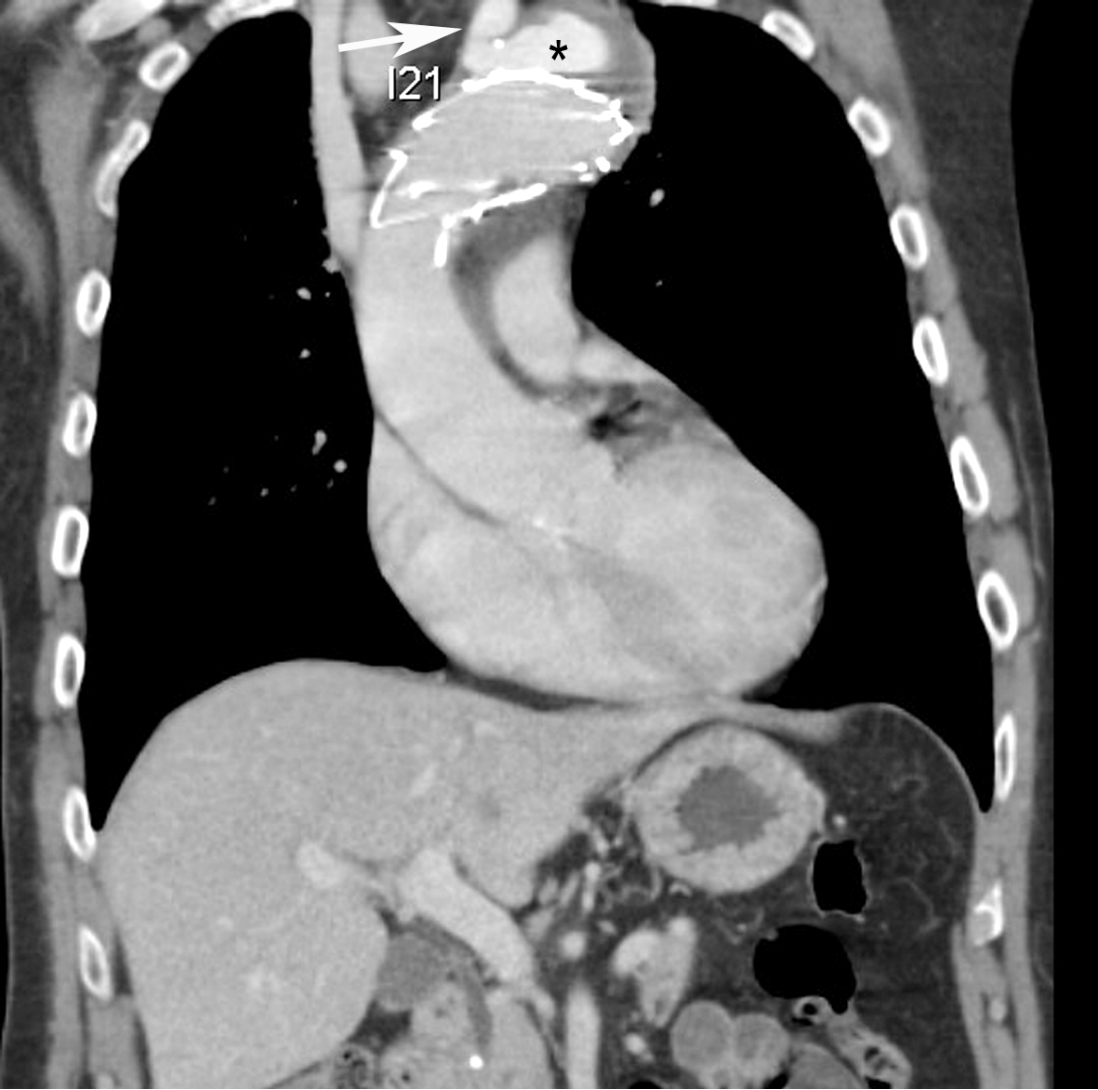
Coronal CT-reconstruction image one year after stent-grafting shows persistent opacification of both the left subclavian artery (white arrow) and the false thoracic aortic lumen (black asterisk).
